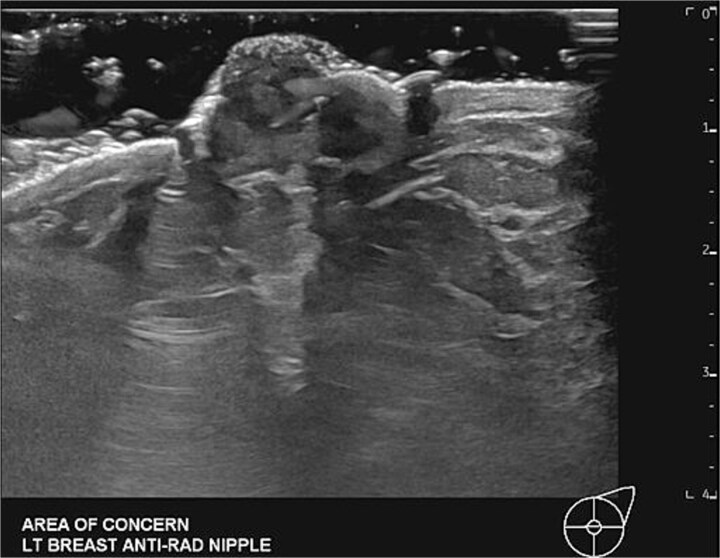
Targeted diagnostic left breast ultrasound demonstrating an enlarged left nipple and heterogenous with diffuse asymmetrically increased vascularity.
